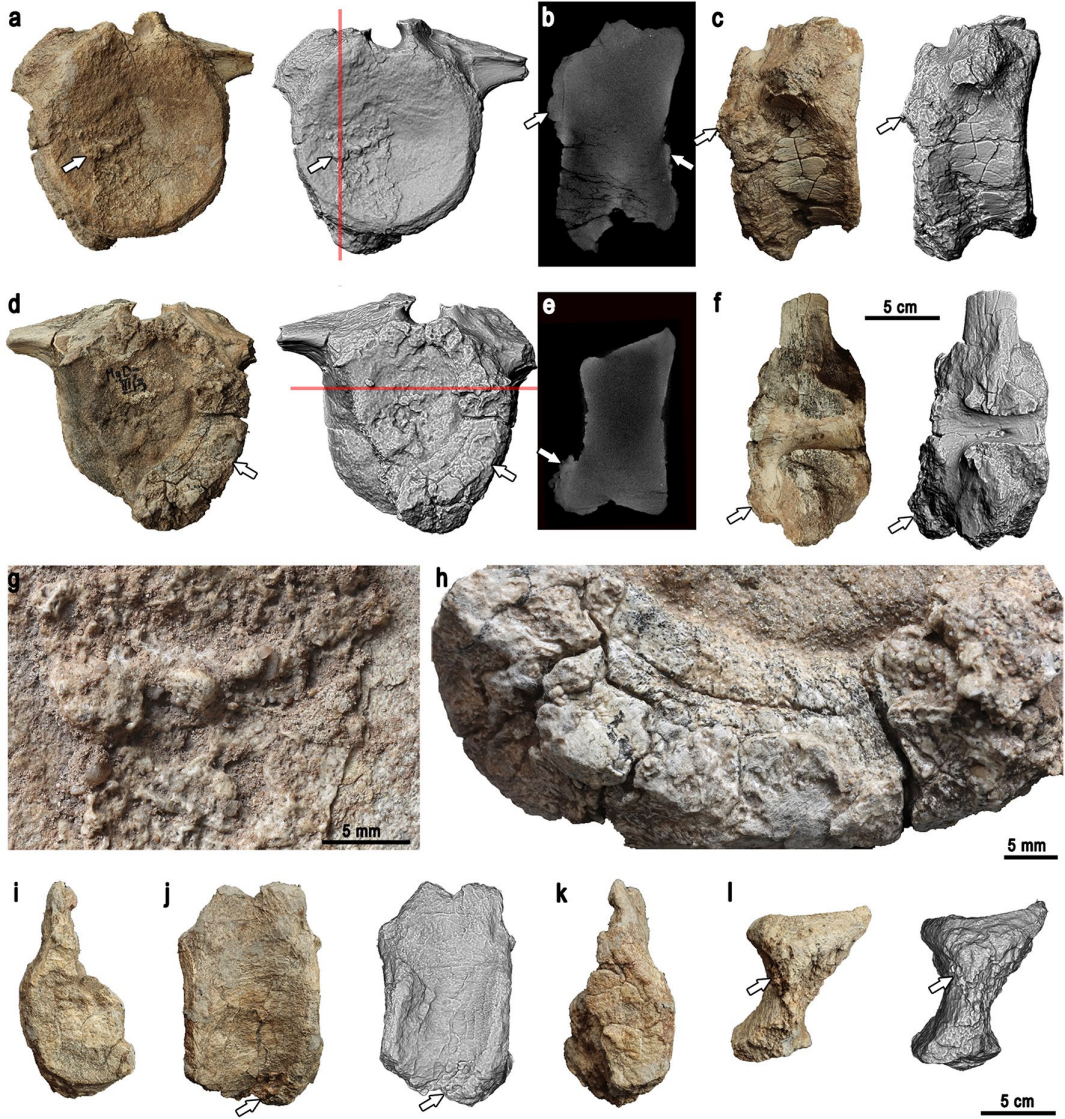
Pathological caudal vertebrae. (a–h) Proximal caudal vertebra. (a) Cranial view, red line indicating the plane of the CT section. (b) Sagittal CT section. (c) Right lateral view. (d) Caudal view, red line indicating the plane of the CT section. (e) Coronal CT section. (f) Dorsal view. (g–h) Closeups of the calcium deposit on the cranial (g) and caudal (h) surface. (i–l) Middle caudal vertebra. (i) Cranial view. (j) Right lateral view. (k) Caudal view. (l) Ventral view. White arrows indicate calcium deposit.
